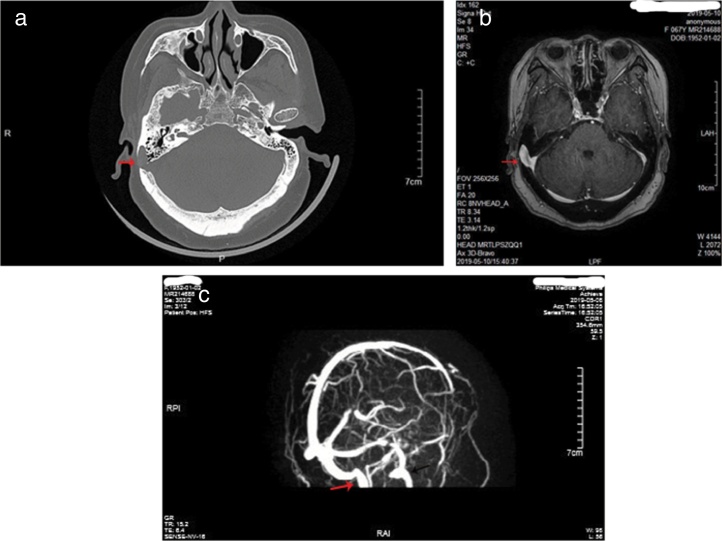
3DCT of the temporal bone revealed an intramastoid diverticulum originating from the right sigmoid sinus (a, red arrow). Enhanced MRI of the brain revealed intramastoid diverticulum of the right sigmoid sinus (b, red arrow). MRV revealed expansion of the sigmoid sinus, transverse sinus, and IJV on the right side compared to the left side (c, red arrow shows right IJV, black arrow shows left IJV).
